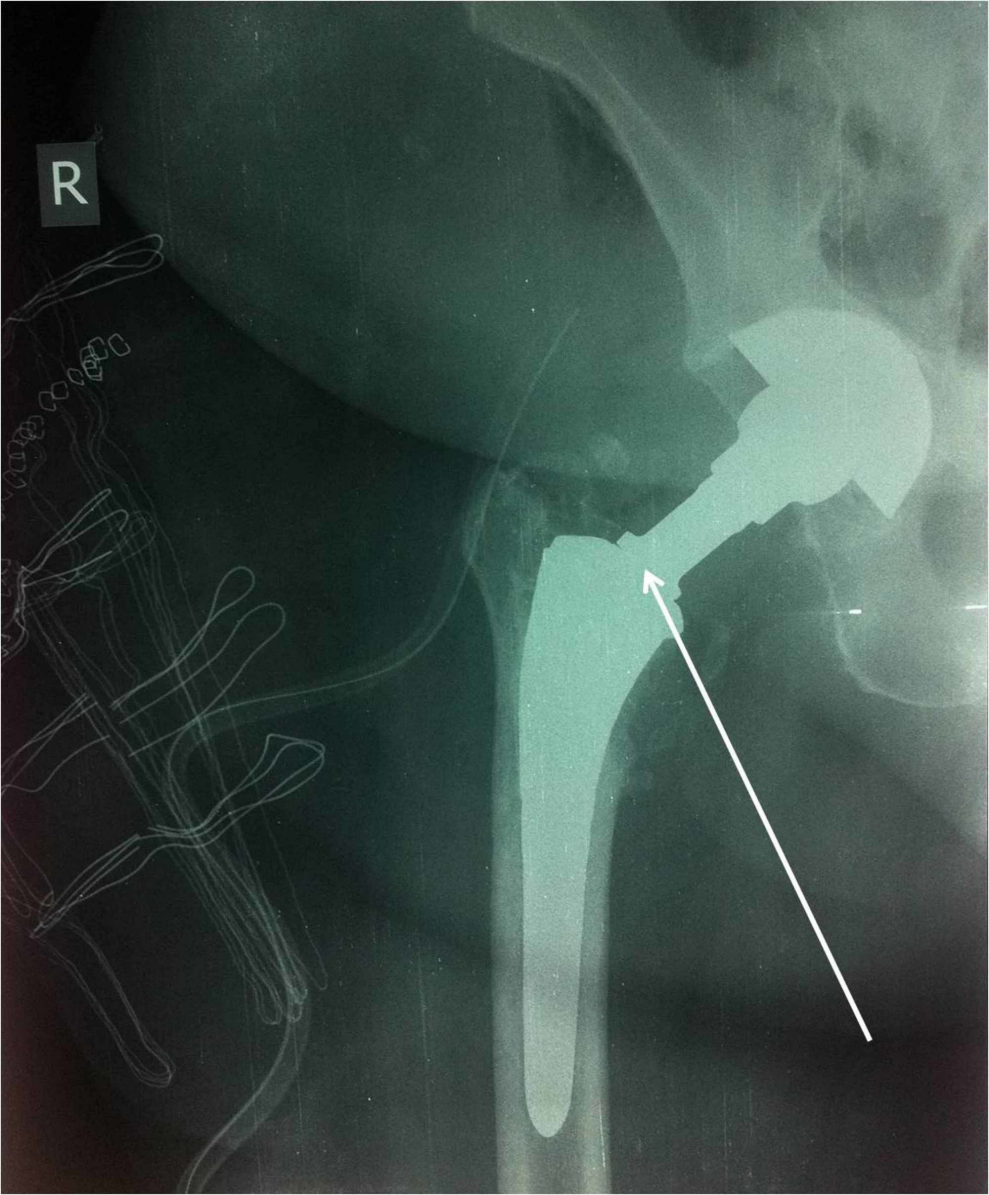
Radiograph after open reduction. Stability test in the neck-stem junction (white arrow) of the modular stem revealed that the prosthesis was stable.
